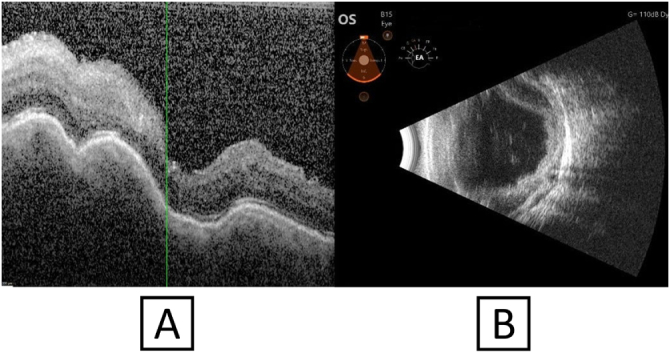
Multimodal imaging of hypotony-related posterior segment changes following ripcord removal from a Paul glaucoma implant. (A) Macular optical coherence tomography of the left eye, showing macular folds associated with hypotony. (B) B-scan ultrasonography of the left eye, showing 360° choroidal detachment.
